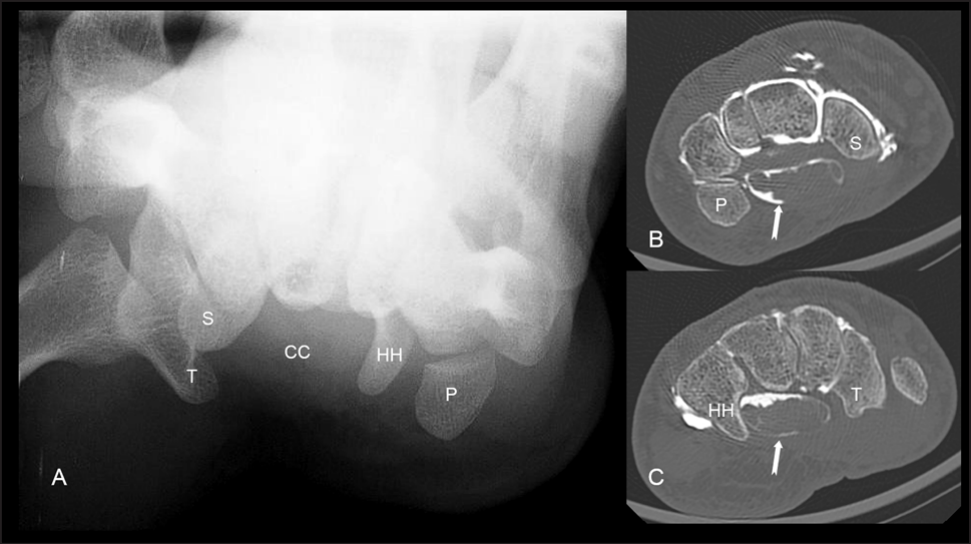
Plain radiography (A, carpal tunnel view in hyperextension) and axial CT views at the level of scaphoidvv/pisiform B) and at the level of trapezium/capitate C). Sca = scaphoid, Pi = Pisiform, Tra = trapezium, Ham = hamate, CC = carpal tunnel. See the opacification of the flexor tendon sheath on the CT arthrogaphy (after injection in the mediocarpal joint).
